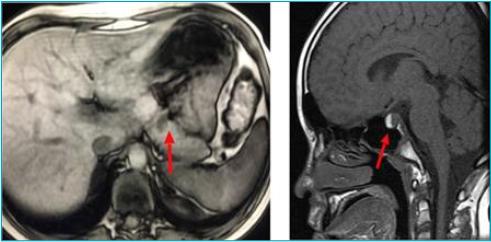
Magnetic resonance imaging of the A) abdomen: pancreatic nodule with hyposignal and well-defined limits (1.0 × 0.8 cm) in the T1out phase sequence without contrast – insulinoma; and B) sella turcica: lesion (10 × 12 mm) with hypersignal suggesting bleeding in the anterior compartment of the adenohypophysis in the T1 sequence without contrast – macroadenoma.
